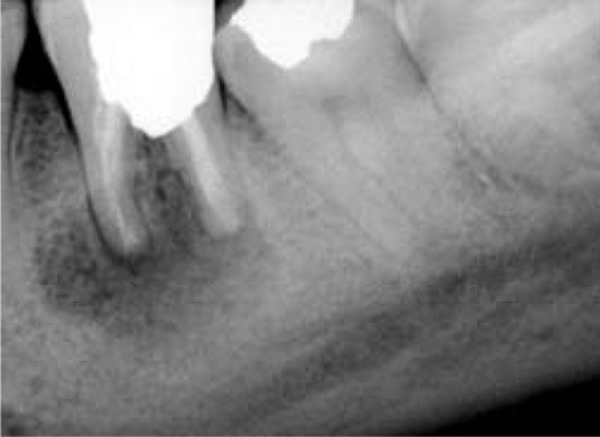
Immediate postoperative radiograph of the replanted tooth
